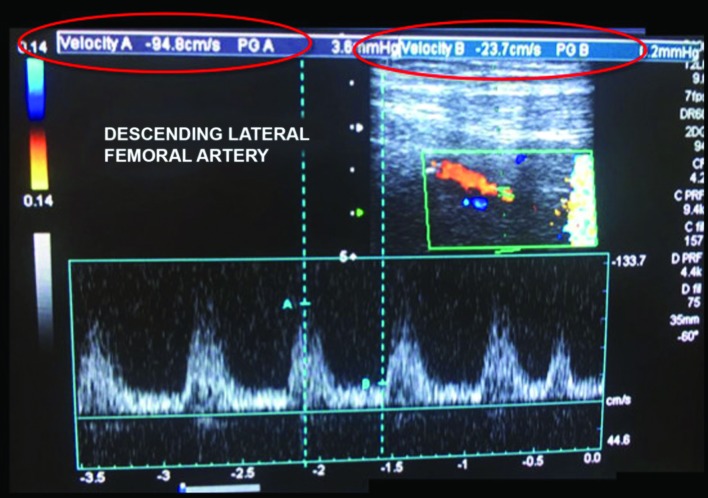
Postoperative follow-up control ultrasound at 5 months, showing adequate outflow via the descending lateral femoral artery, with biphasic wave, normal acceleration, and good amplitude.
